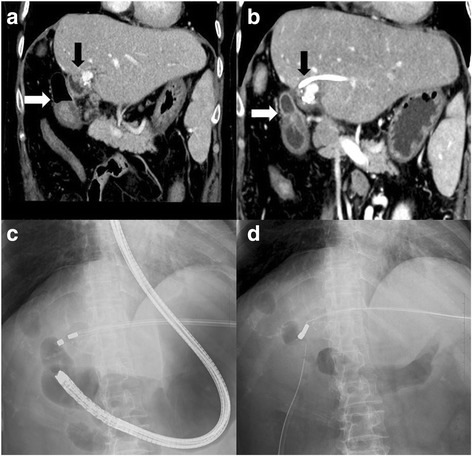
a Computed tomography showed the bulbus duodeni (*white arrow*) and biliary duct (*black arrow*) come in contact with each other. b PTBD tube was placed into B3. c The parent magnet was inserted from PTBD tube, and daughter magnet was placed by gastrointestinal endoscopy. d The parent and daughter magnets connected sandwiching the biliary wall and duodenal wall
